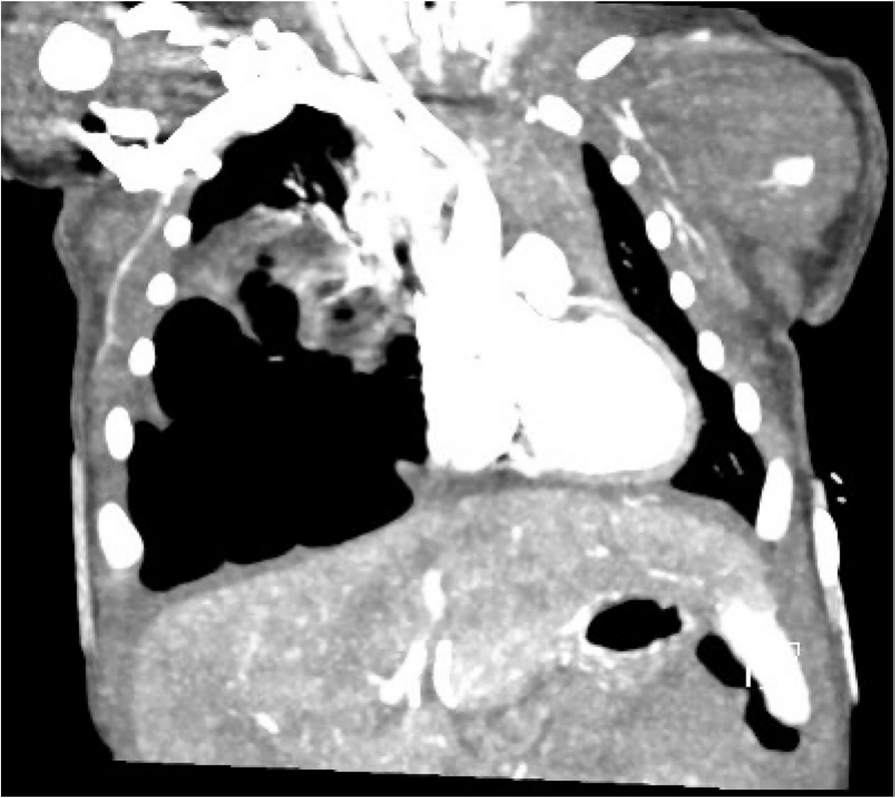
Chest computed tomography angiogram demonstrates a macrocystic multicystic lesion within the right lower lobe without systemic arterial supply
